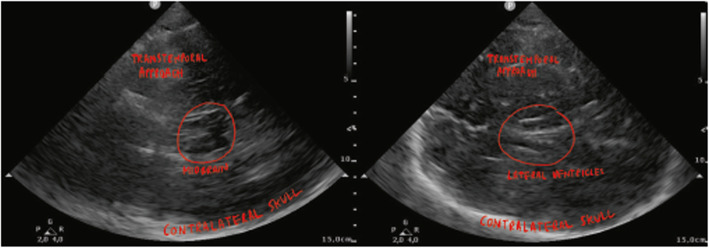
Left panel: Midbrain as the main landmark to explore the circle of Willis; once that is found, the power Doppler can be started to scan for intracranial arteries. Right panel: Lateral ventricles in a severe TBI patient with pronounced midline shift and trans-tentorial herniation
